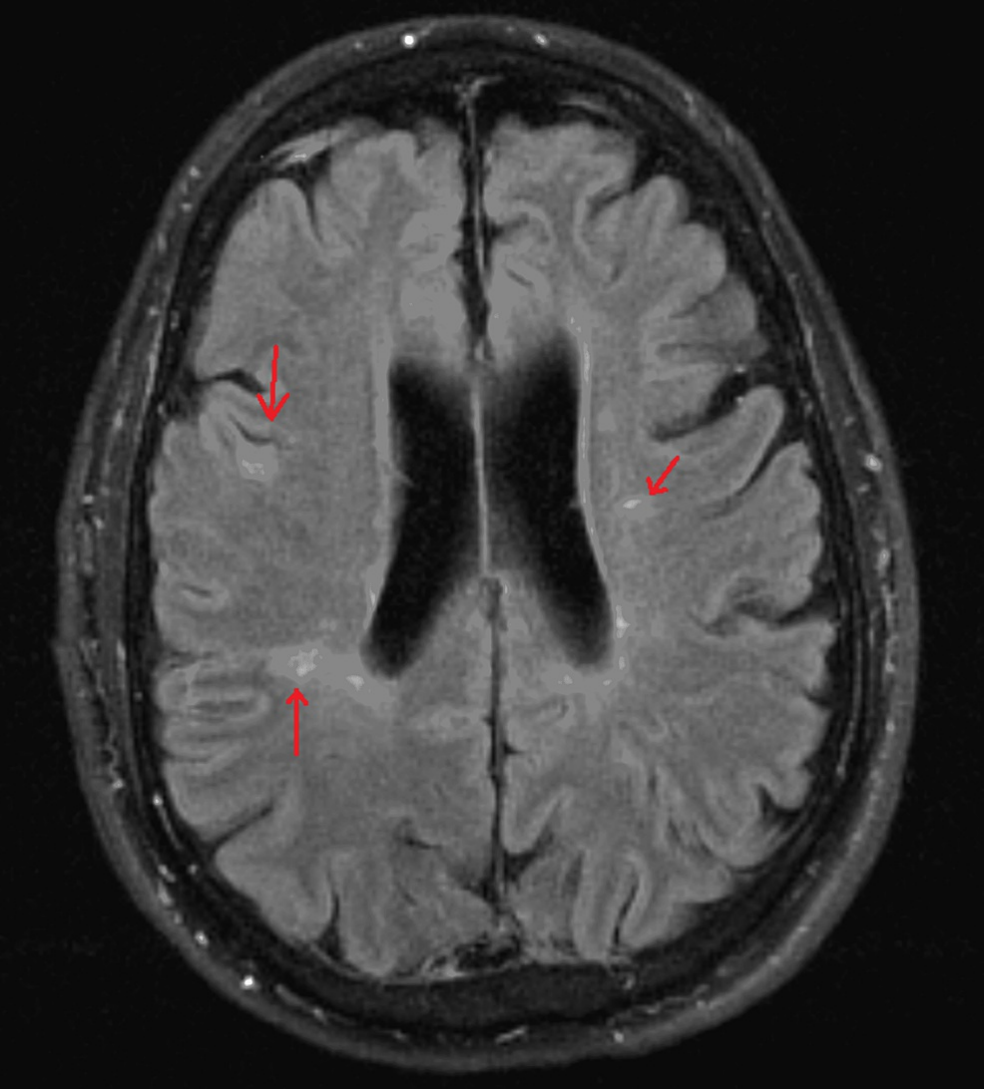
Bilateral T2-FLAIR periventricular and deep white matter signal abnormalities seen on the axial image of the brain, which given the patient's clinical history, are consistent with chronic demyelinating disease Signal abnormalities are indicated by the red arrows. FLAIR - fluid attenuated inversion recovery
